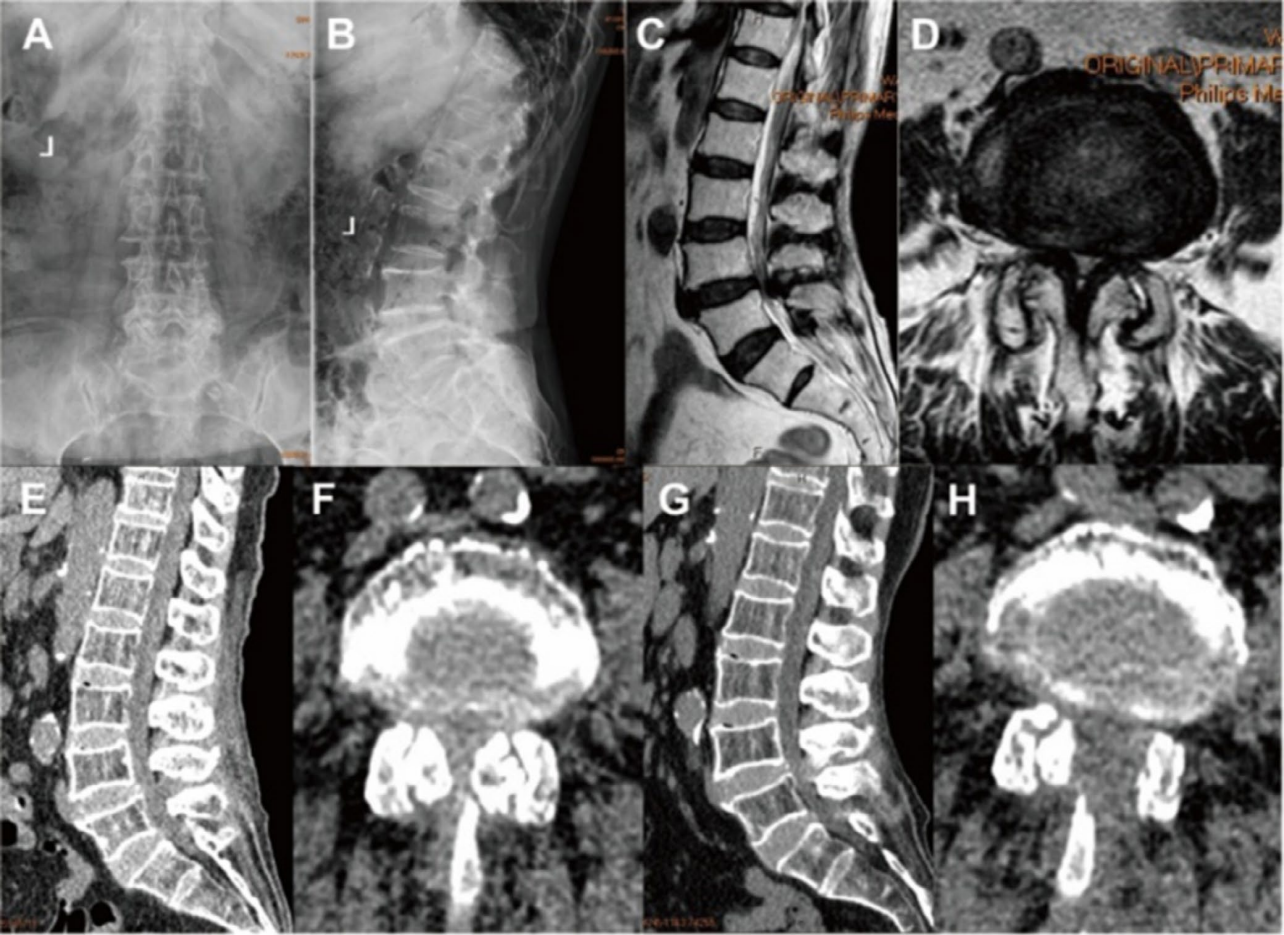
Radiological images of UBE technique under LA. (A, B) Preoperative AP and lateral view X rays showed obvious degeneration at the L4/5 level. (C–F) Preoperative MRI and CT scans showed stenosis at the L4/5 level. (G–H) Postoperative CT scans showed sufficient decompression after UBE decompression. AP, anterior–posterior; CT, computed tomography; LA, local anesthesia; MRI, magnetic resonance image; UBE, unilateral biportal endoscopy.
